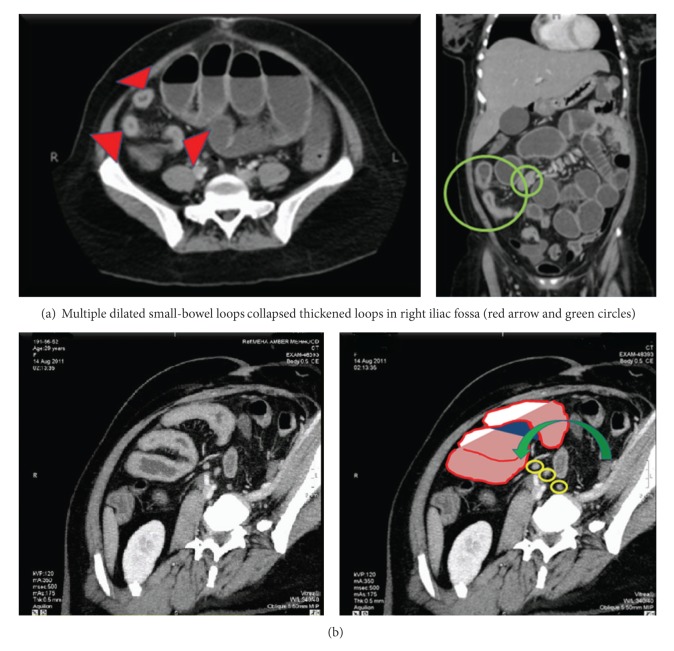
CT scan of 48-year-old female with acute abdominal pain. (a) Axial and coronal CT images obtained with intravenous contrast agents show dilated small-bowel loops (red arrow heads and green circles). (b) Oblique reformation shows dilated small-bowel loops with a transition point (green arrow) in the midabdomen. Multiple small mesenteric lymph nodes also visualized (yellow circles).
